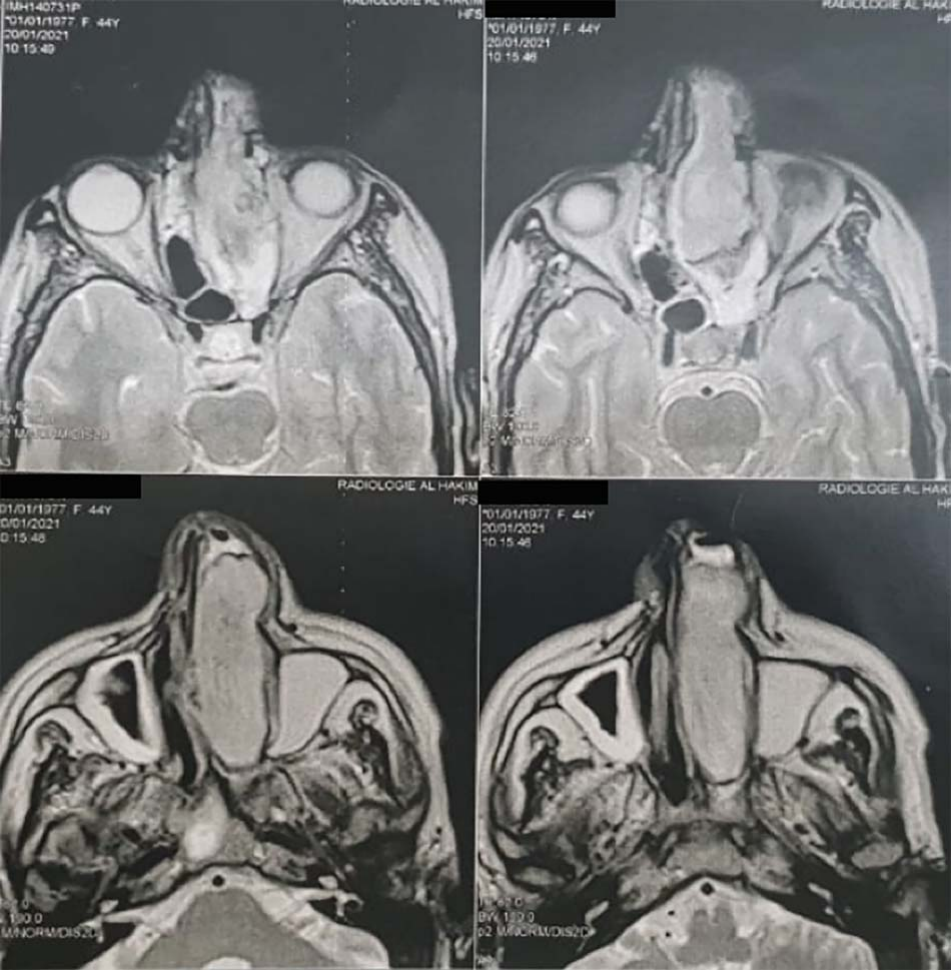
Head MRI showing a filling of the left nasal cavity by a neoplastic process.
